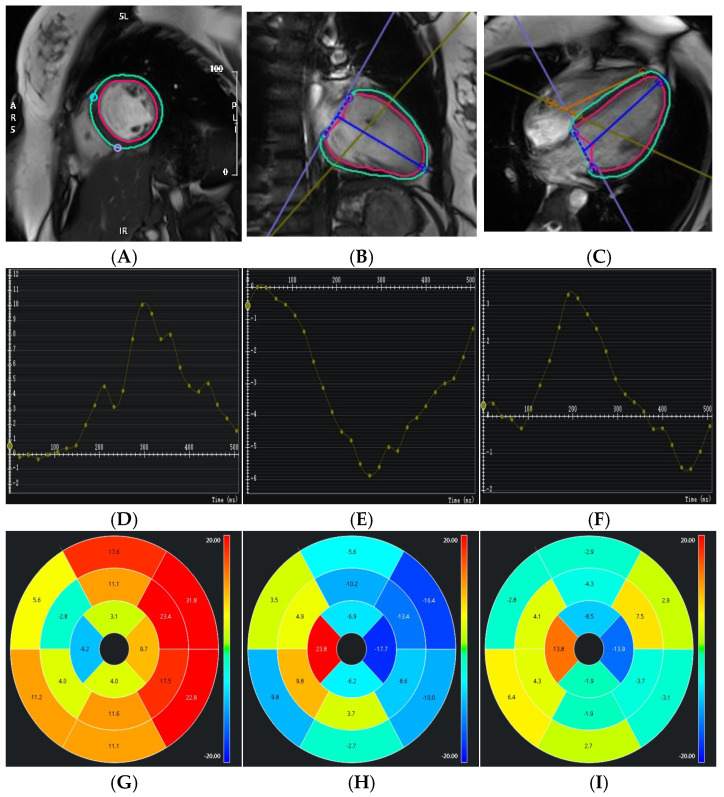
Example MRI studies of a 23-year-old man with NDLVC-rLVEF who was hospitalized for heart failure 8 months after the cardiac MRI examination. (A–C): Left ventricular strain images in the short-axis, two-chamber, and four-chamber views, respectively. The contours of the left ventricular endocardium (red) and epicardium (green) are delineated. (D–F): Strain curves for 3D left ventricular global radial strain (GRS), global circumferential strain (GCS), and global longitudinal strain (GLS), respectively. (G–I): Sixteen-segment bull’s-eye maps displaying the distribution of GRS, GCS, and GLS, respectively.
